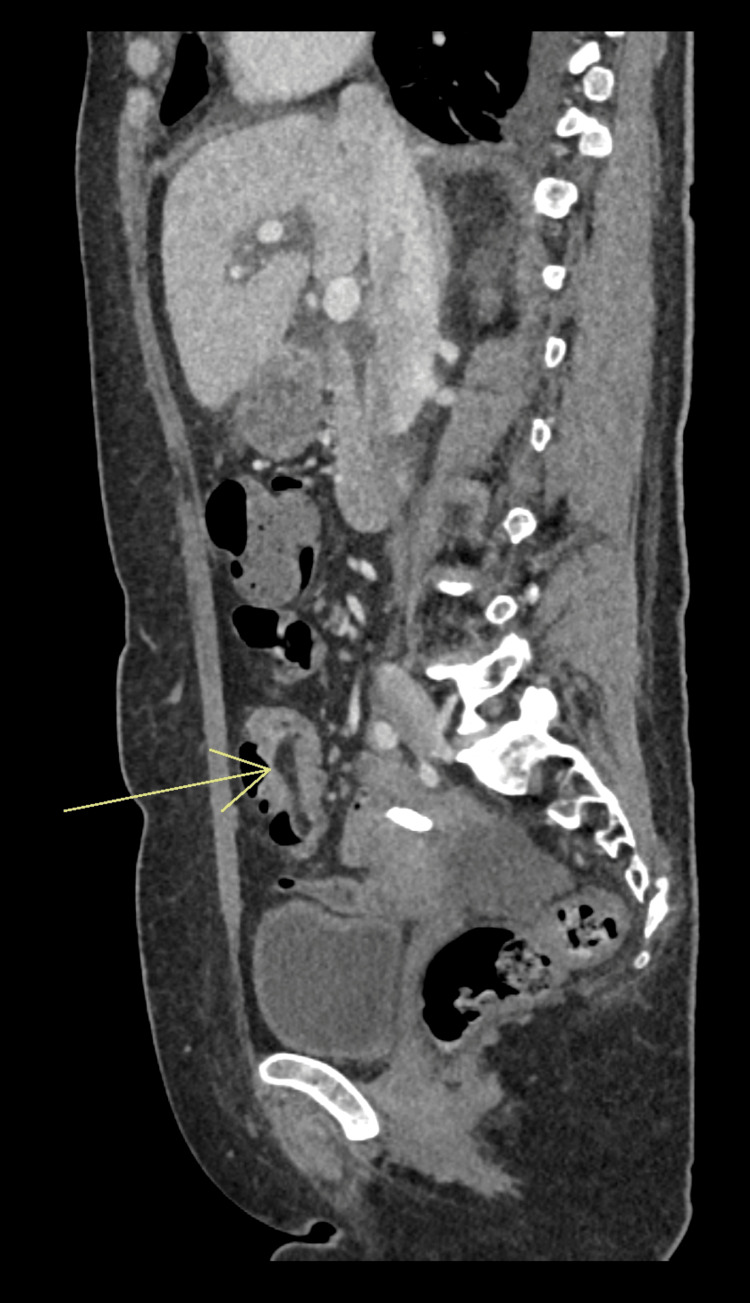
Sagittal view of the polyp shown on CT scan abdomen with contrast
